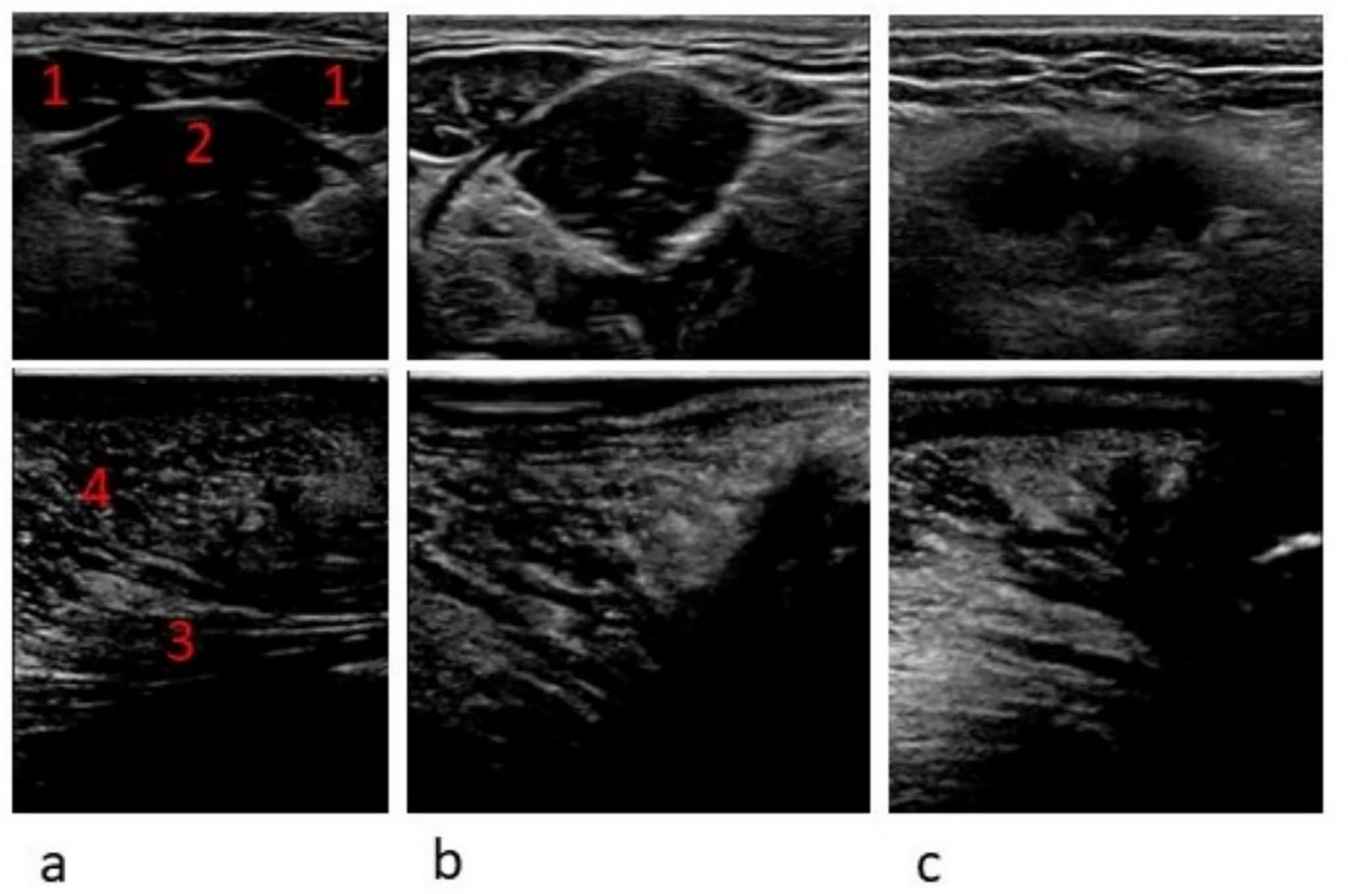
Clear (a), questionable (b) and unclear (c) ultrasound images of both digastric muscles (1), the geniohyoid muscle (2), the genioglossus muscle (3) and the transverse muscle (4)
